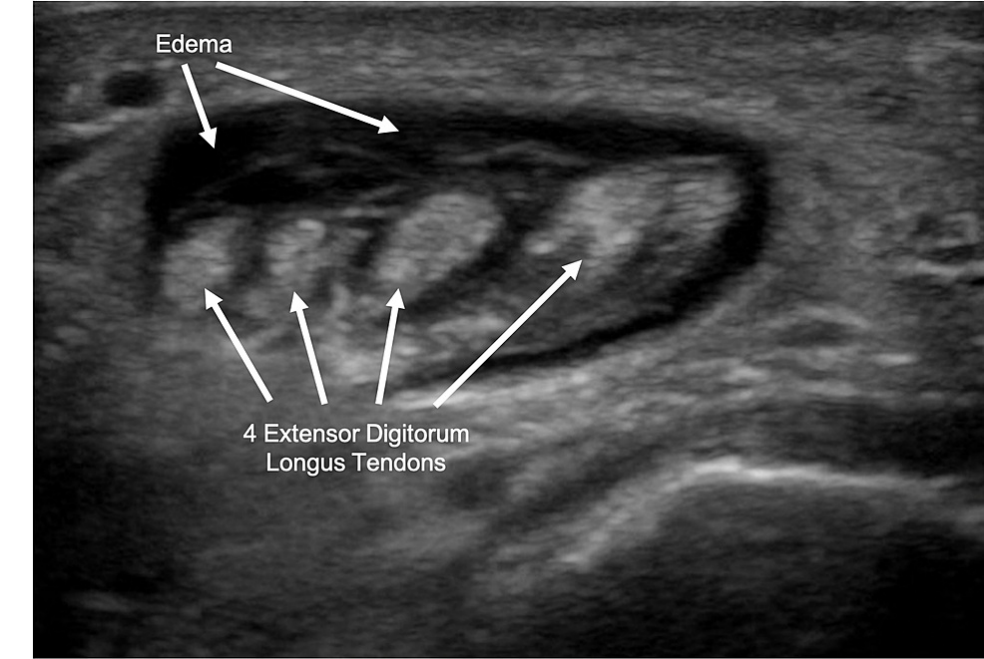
Transverse ultrasound showing soft-tissue fluid collection with hyperechoic structures in the area of extensor digitorum tendons
